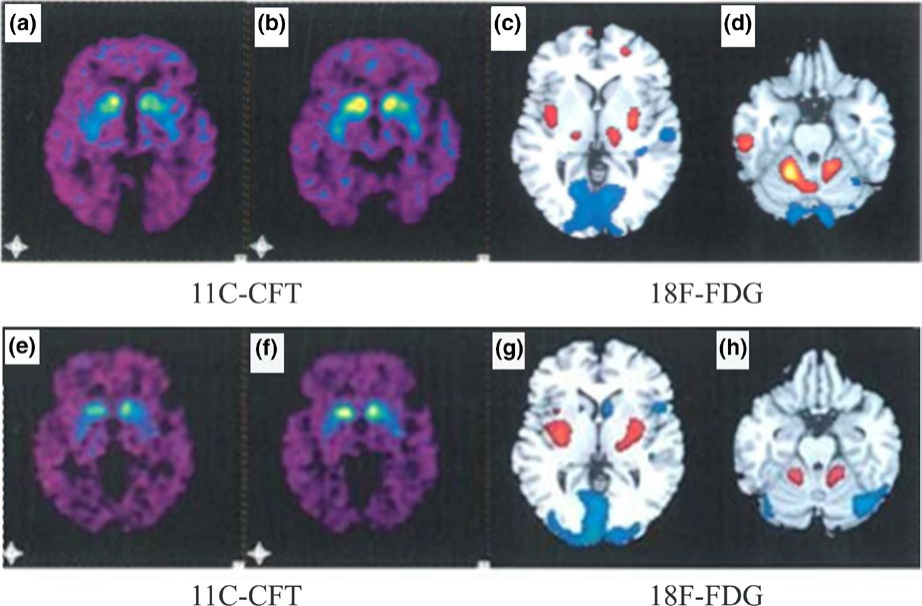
11C‐CTF and 18F‐FDG PET/CT brain images of II‐2 (a‐d) and II‐7 (e‐g)
